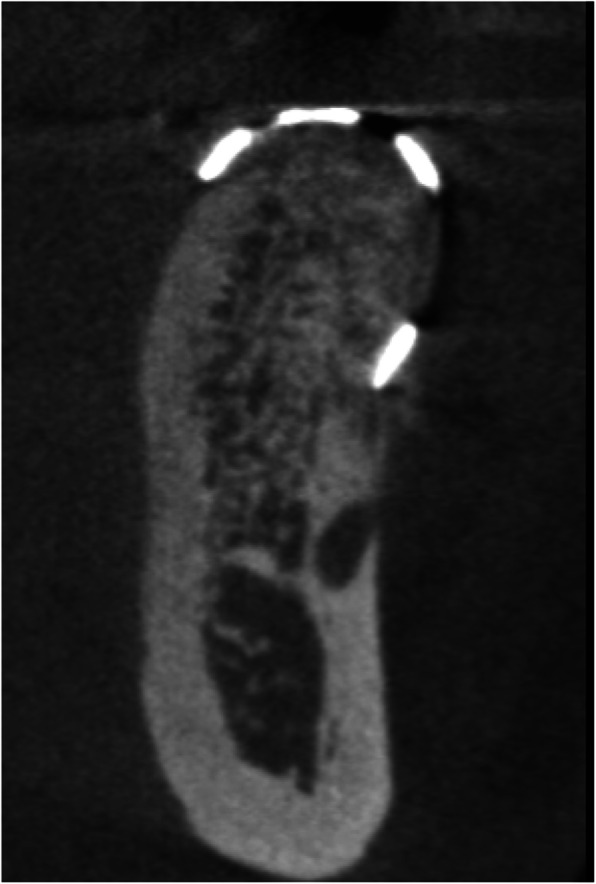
CBCT 7 months post-operatively, suggesting complete bone fill
